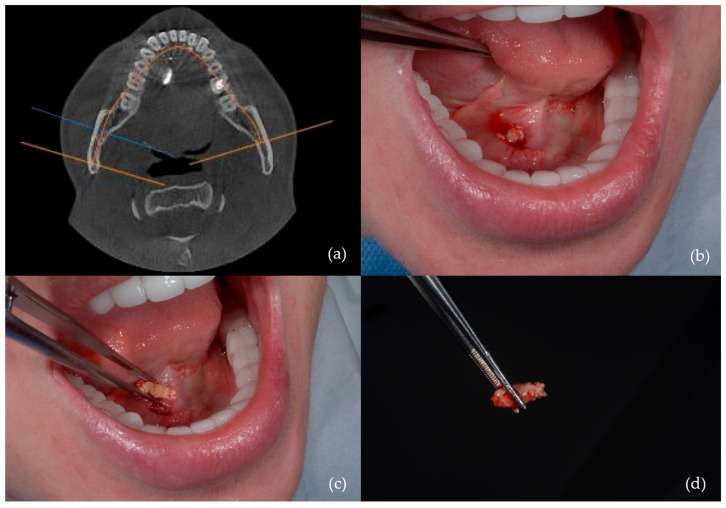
Sialolithotomy of a 9 mm calculus: (a) CBCT axial view showing the radiopaque calculus; (b) intraoperative view; (c) calculus removed from Wharton’s duct; (d) the calculus following sialolithotomy.
